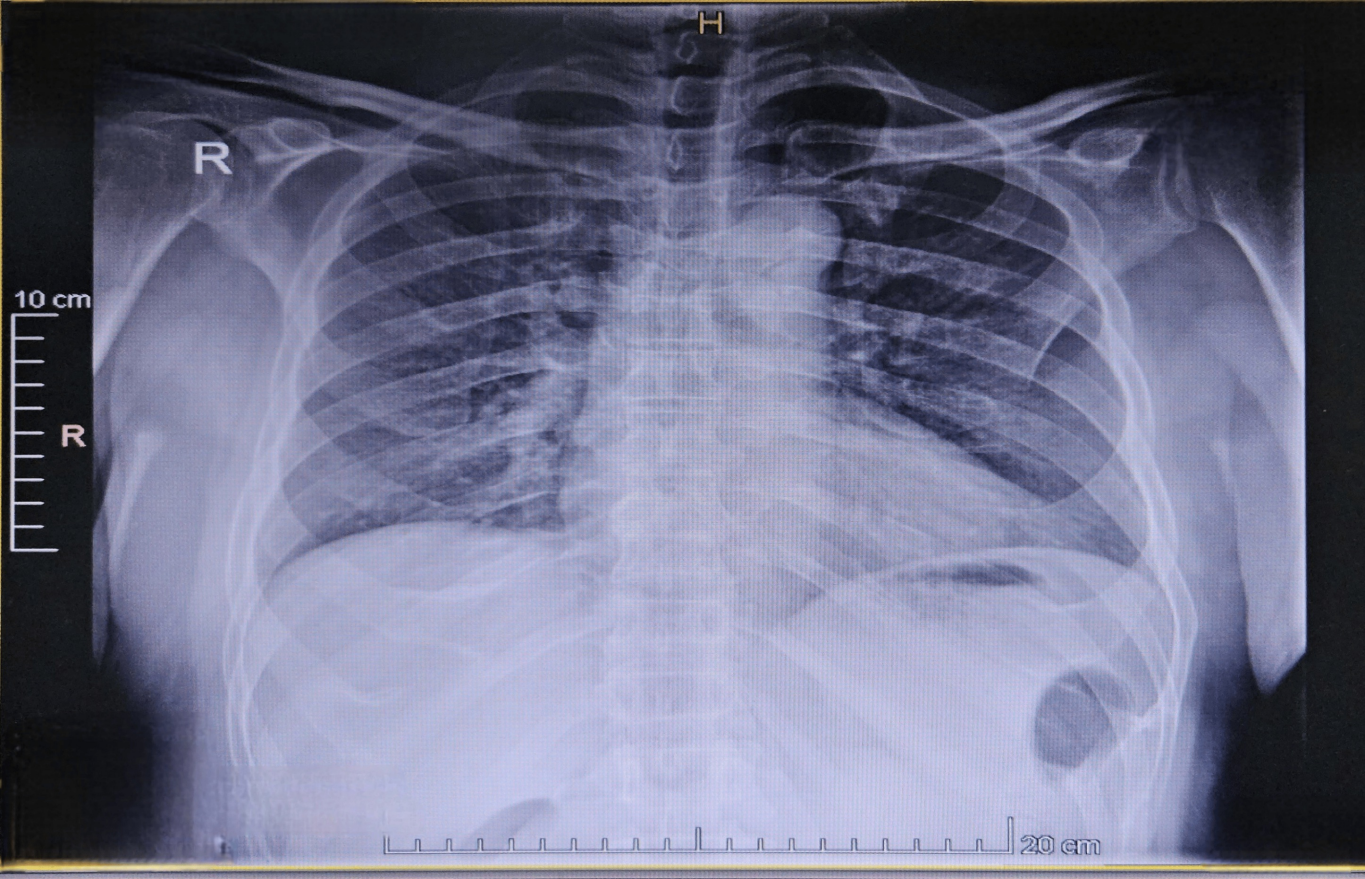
Chest X-ray with no significant finding.
